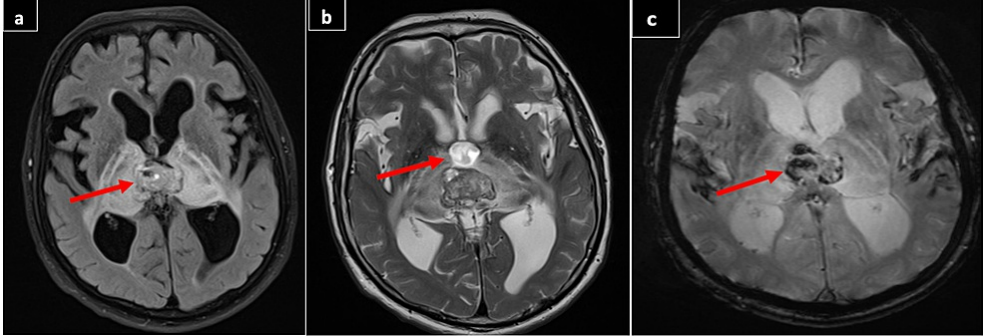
a) MRI of the brain with contrast axial view showed increased in the size of previous pineal region mass 3x2x3.5 cm with marked contrast enhancement and extensive edema (arrow). The mass and edema are extending bilaterally with thalamic compression, marked posterior third ventricular obstruction and marked active obstructive hydrocephalus. b) MRI T2 weighted image showing the same mass with heterogenous intensity (arrow). c) MRI of the brain flair view showing the same mass heterogenous signal intensity with multiple foci of low signal indicating hemorrhagic component (arrow).
